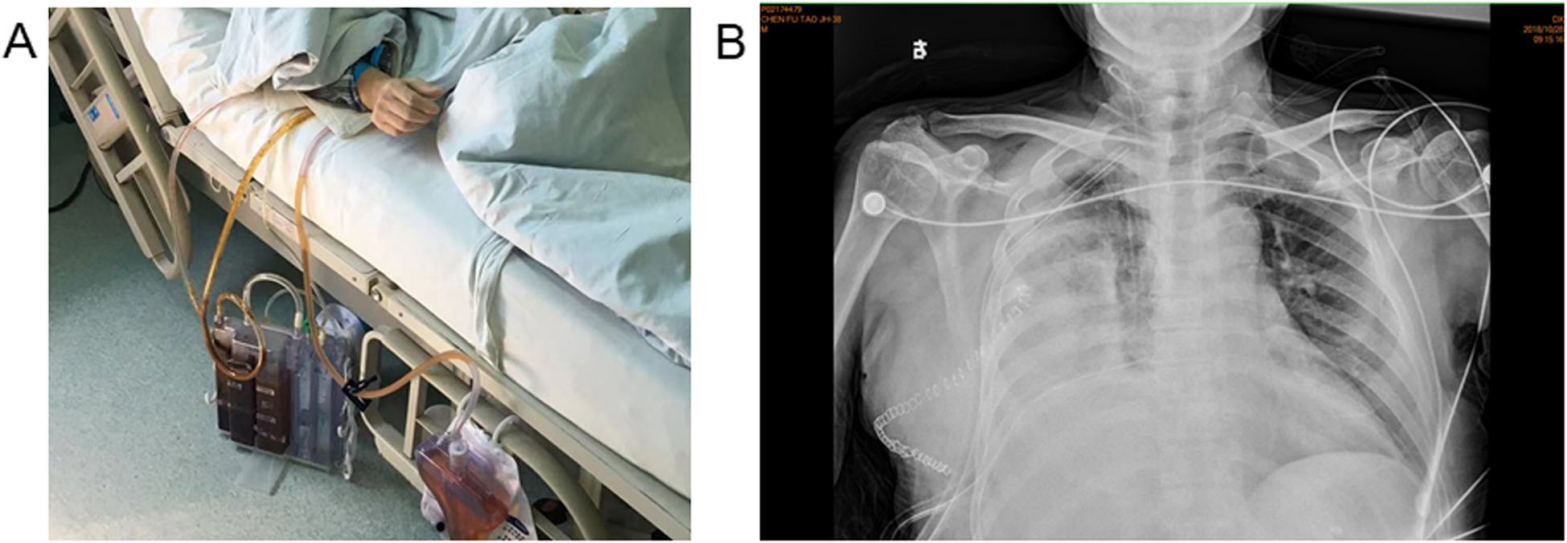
Postoperative empyema. a turbid stool like fluid drained out from the chest tube. b chest X-ray exhibited decreased transparency in the right chest cavity
